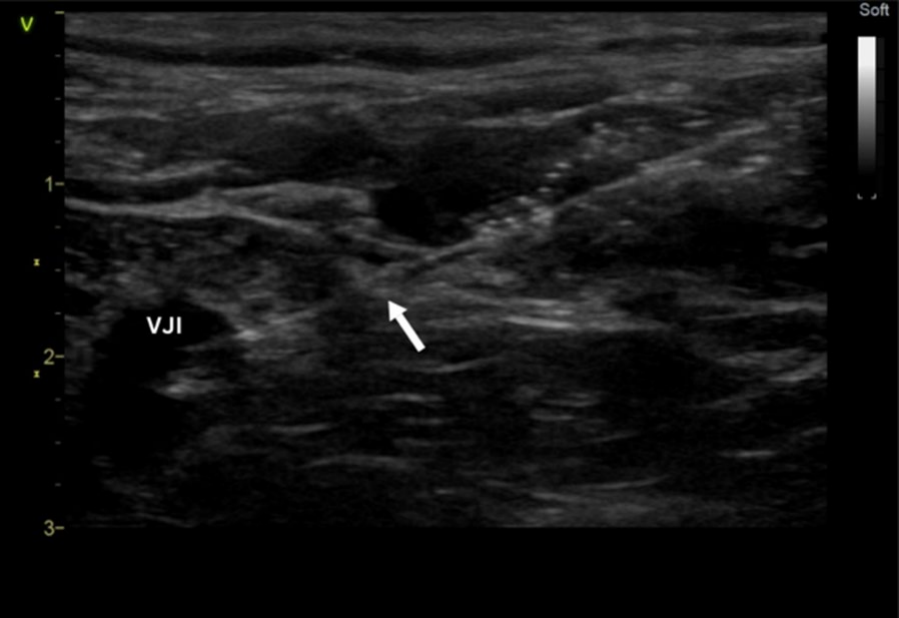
Ultrasound image visualizing needle (white arrow) in-plane as hyperechogenic line entering an IJV
